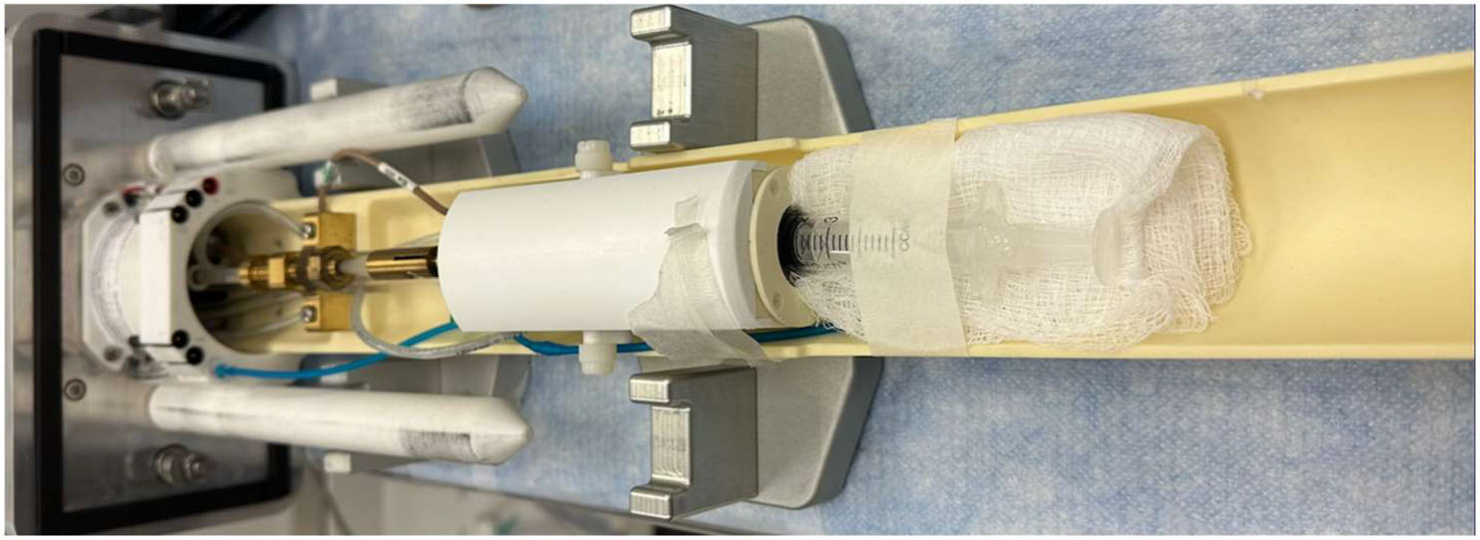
Securing the sample‐containing syringe to the 23‐mm‐diameter MRI coil. Gauze can be used to elevate the sample to the appropriate height, and paper tape is placed across the top of the syringe to prevent any movement.
